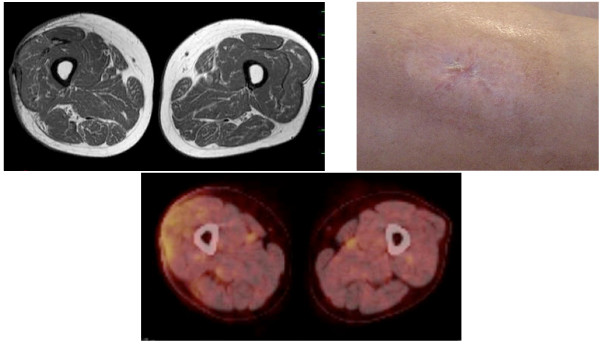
Coronary sections of MRI and PET-CT, and Photograph on the right thigh. No tumor exist 30 months after treatments on these images.
